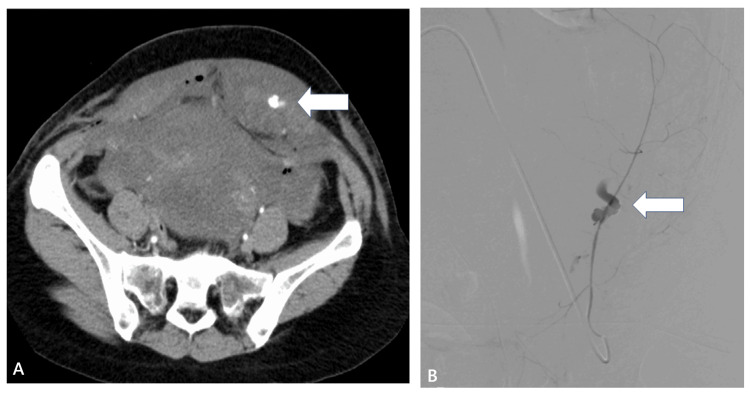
Radiologic images 38-year-old woman with bleeding within 24 hours after a cesarean delivery. (A) A contrast-enhanced CT shows active contrast extravasation in the left abdominal wall (arrow). (B) A left inferior epigastric artery angiogram shows active contrast extravasation in the abdominal wall (arrow).
